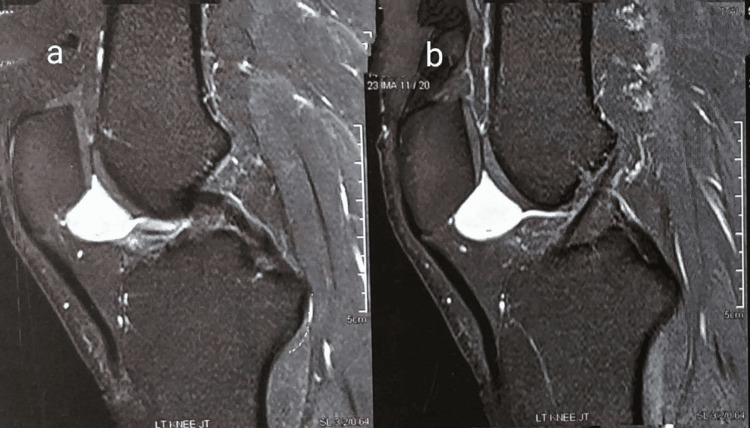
MRI images of the left knee joint A well-defined globular lesion appearing intermediate to hypointense on T1, showing avid enchantment, was noted in the infra-patellar recess within the Hofa's fat pad and abutting the inferior-posterior (non-articular) surface of the patella. It shows a thin linear extension arising from the anterior surface of the Anterior Cruciate ligament - suggestive of neoplastic etiology.
